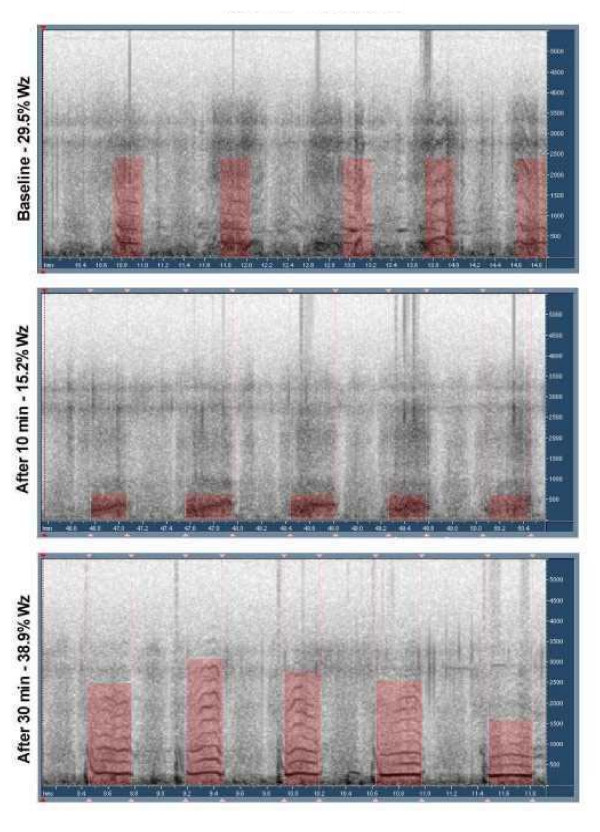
Sonogram of subject treated with albuterol, showing no response.
